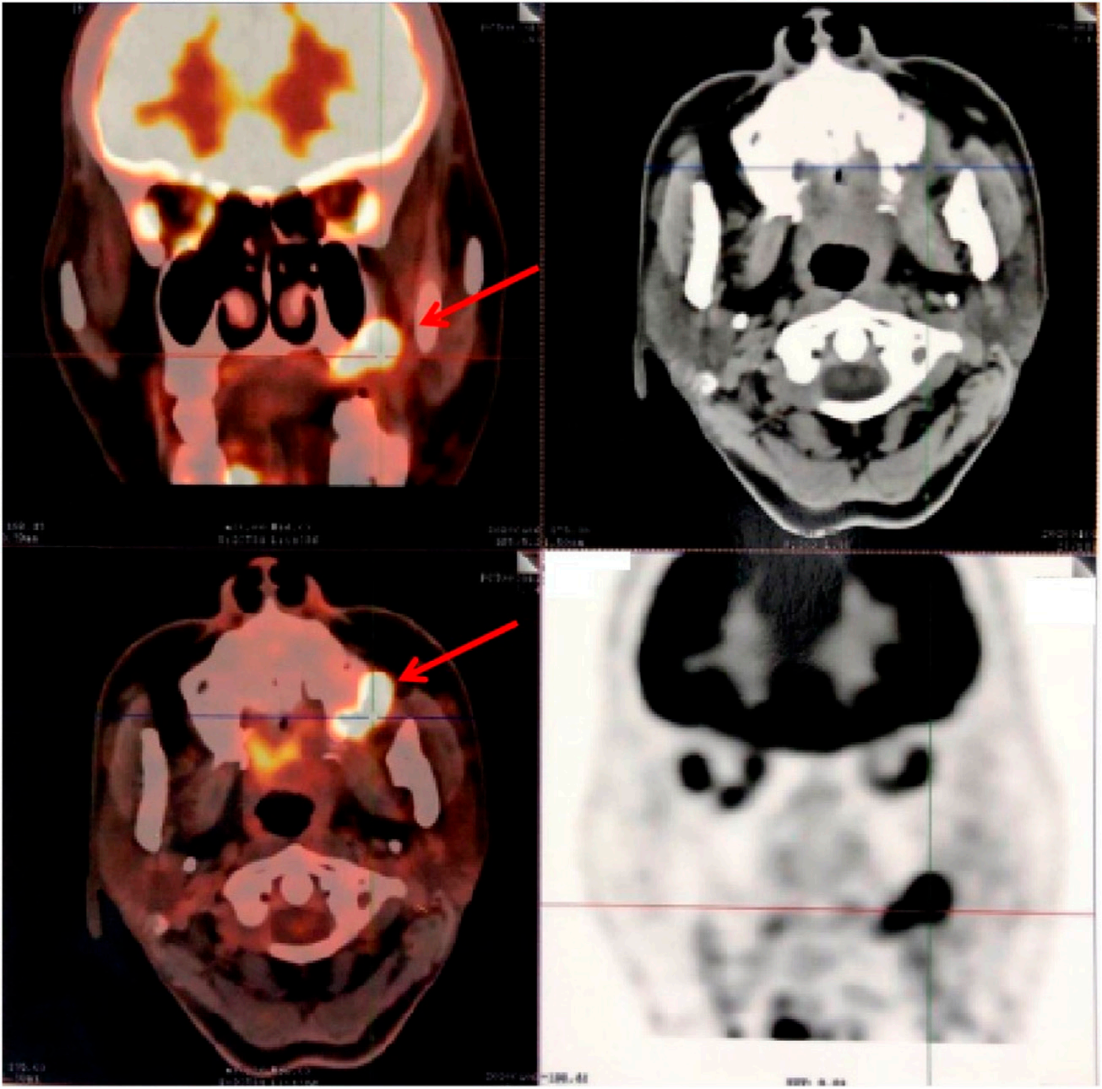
PET-CT axial view shows metabolic hyperactivity of the maxillary mass on the left lateral wall.
